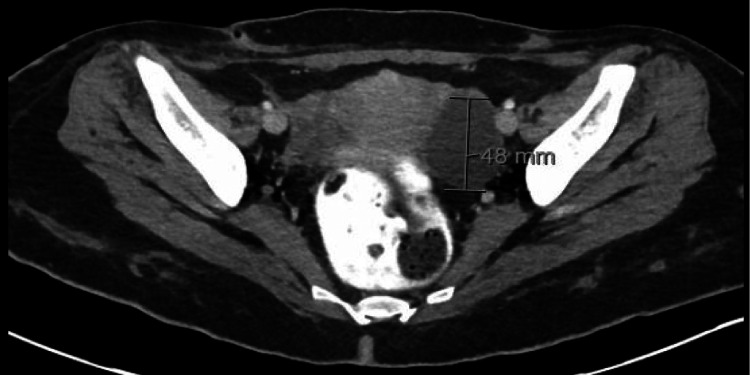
CT abdomen and pelvis with contrast showing a complex 48 mm fluid collection with fistulous tracks within the pelvic cavity.
